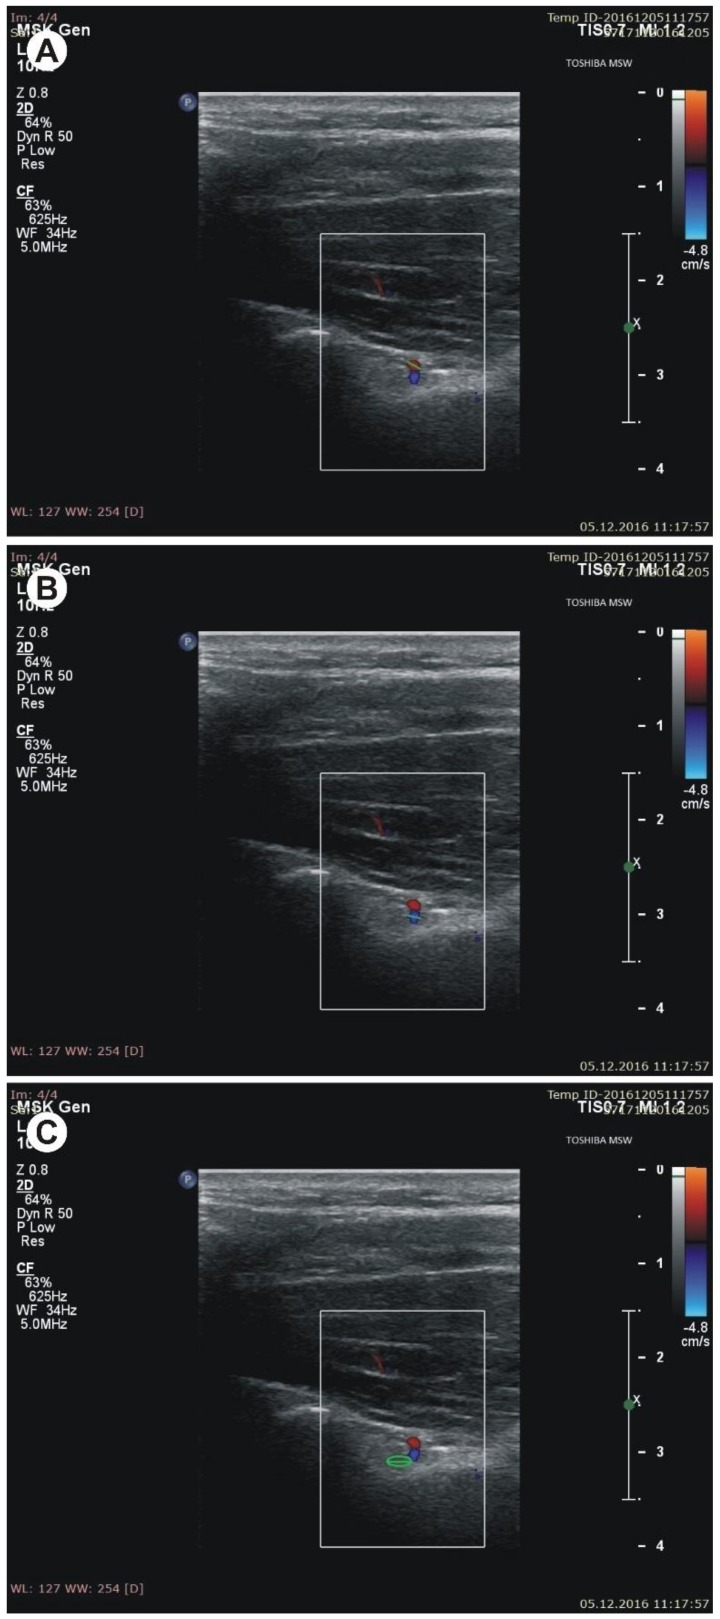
Measurements of structures at the suprascapular notch (SSN) notch region during sonographic examination. (A) diameter of the suprascapular artery; (B) diameter of the suprascapular vein; (C) diameter of the suprascapular nerve.
